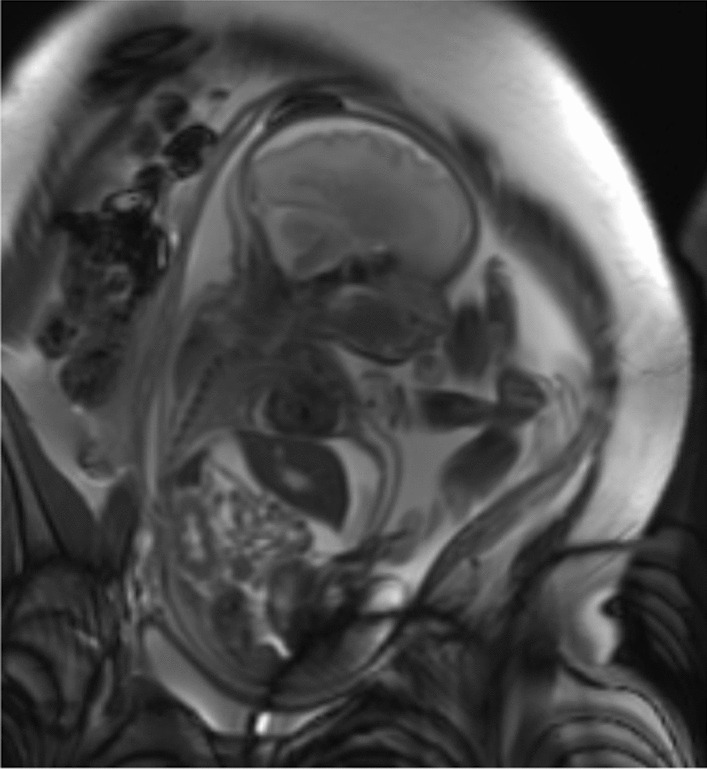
MRI T2 image showing fetal urinary ascites compressing the thoracic cavity, but no signs of pulmonary hypoplasia and normal kidneys
